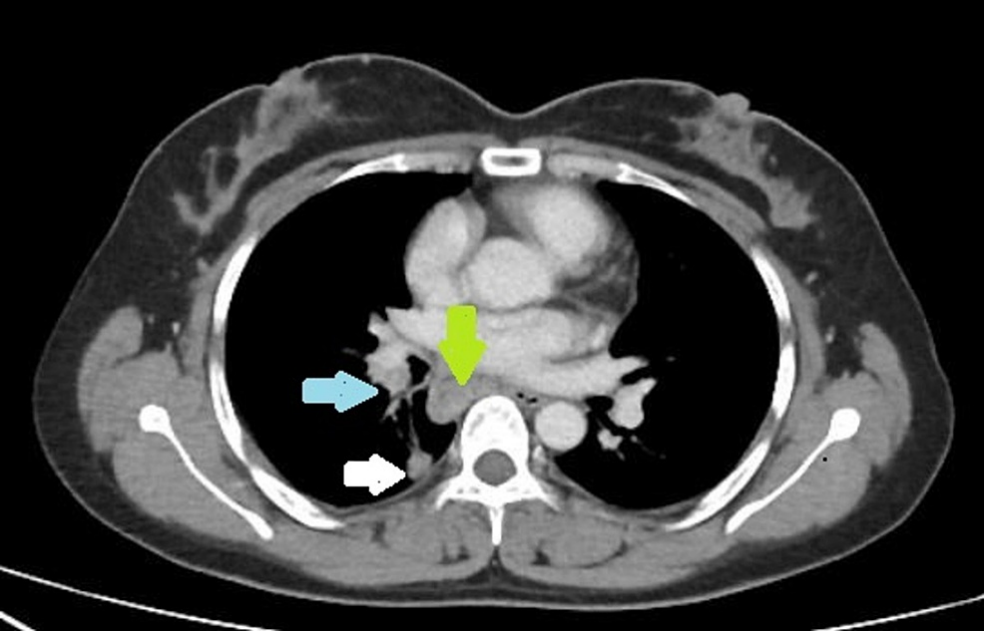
A section from the contrast enhanced CT Chest of a patient diagnosed with adenocarcinoma lung, showing right lower lung nodule (white arrow) and enlarged right hilar (blue arrow) and subcarinal (green arrow) lymph nodes.
